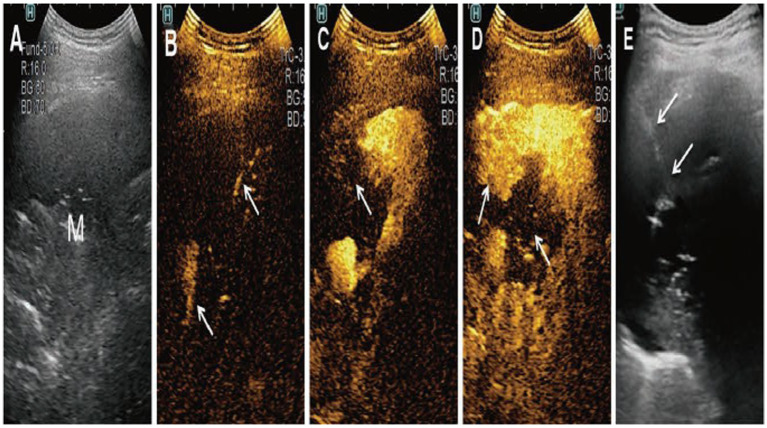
Lung puncture under contrast-enhanced ultrasound. (A) Conventional ultrasound showed a mass lesion (M). (B) Contrast agent reaching lung tissues. (C) Contrast agent reaching lesions tissues. (D) The mass was completely enhanced. The enhancement mode is centripetal, non-uniformly. (E) Ultrasound-guided puncture.
